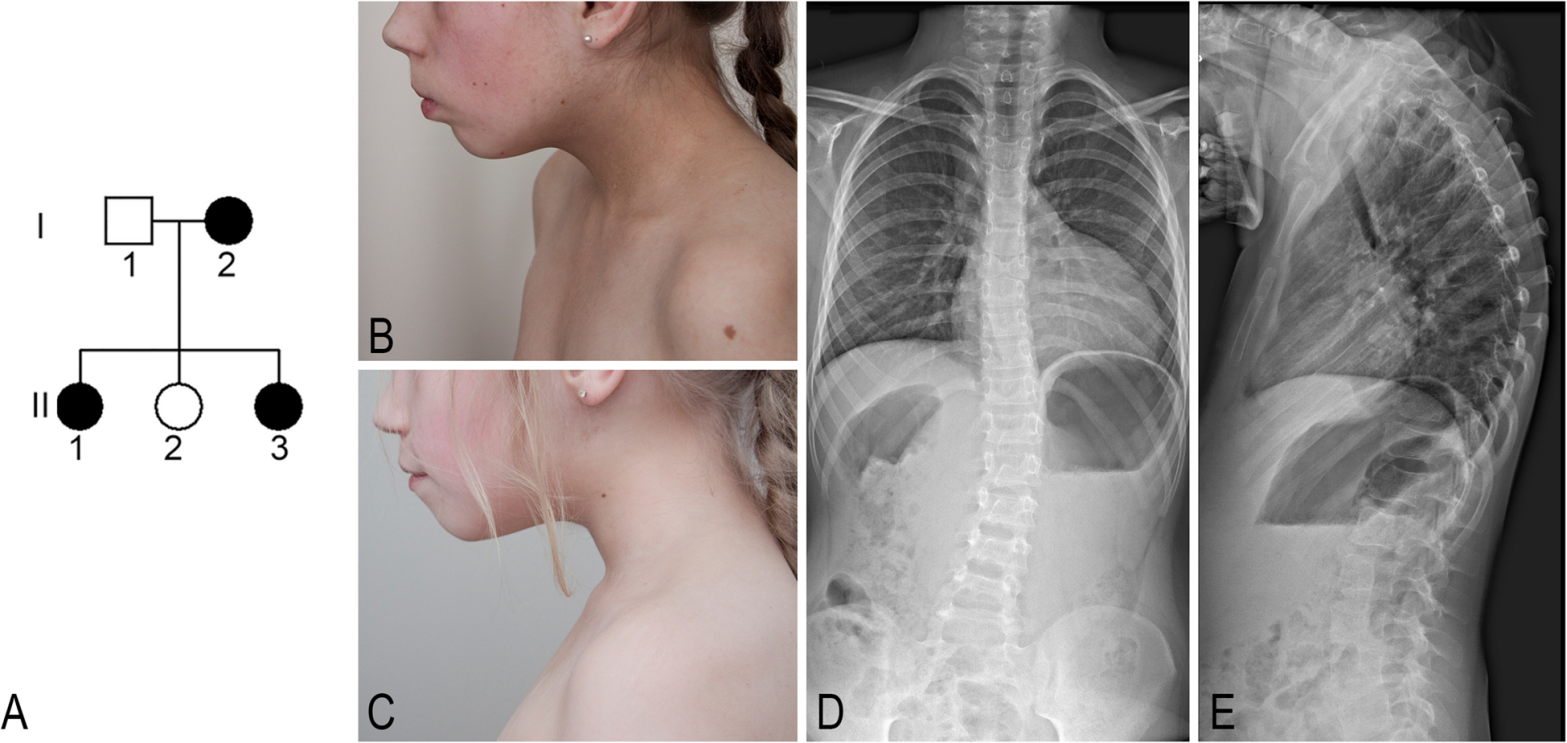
a. The genealogy of the family. The black symbol denotes affected individuals. b. Side facial view of patient 1 at age of 14 years. c. Side facial view of patient 2 at age of 9 years. d-e. Chest X-ray of patient 2 at age of 10 years showes “S” shaped II° scoliosis of the thoracolumbar spine
